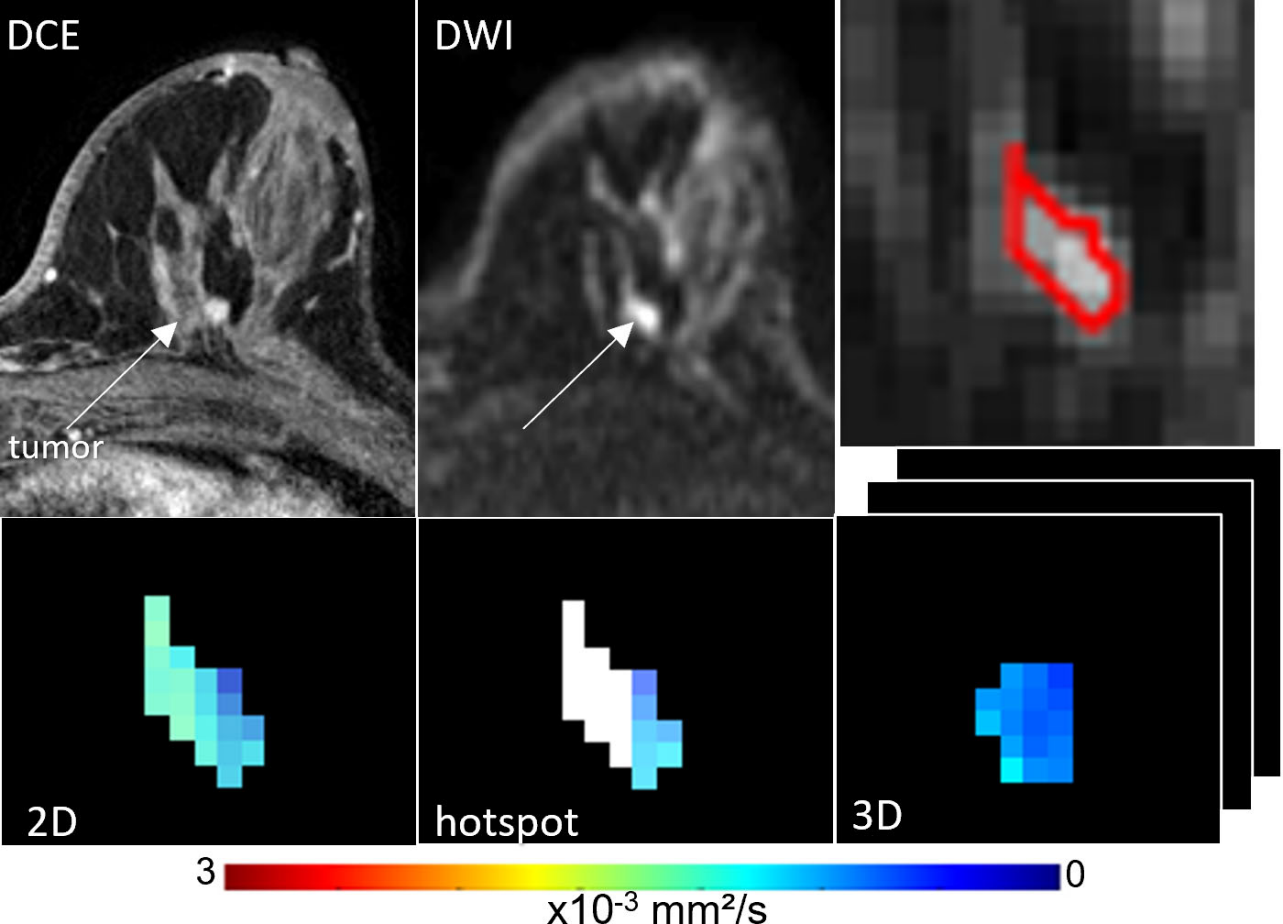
ADC measures of a BI-RADS 4 6 mm mass detected in a 55-year-old woman who underwent screening MRI. Lesion ADCs calculated using the different ROI techniques were 1.21, 1.17 and 1.22 x10^-3^ mm^2^/s for 2D, hotspot, and 3D ROIs, respectively. The lesion was invasive ductal carcinoma on biopsy.
